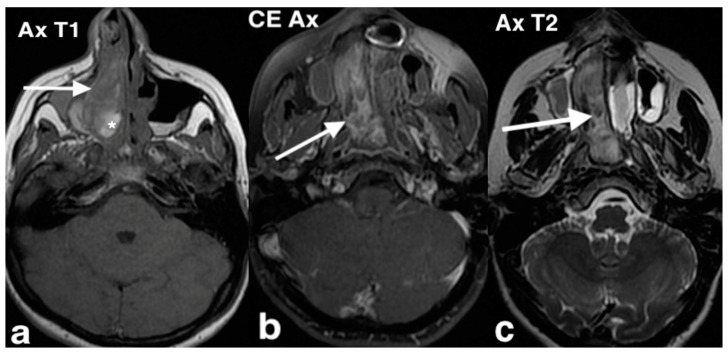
Hemangiopericytoma in a woman with epistaxis and bilateral nasal obstruction. On axial T1 MR image (a), the right nasal cavity is entirely occupied by an obstructing mass (arrow) with low–intermediate intensity and hyperintense hemorrhage areas (asterisk). Posteriorly, the mass, crossing the right choana, obstructs the nasopharynx lumen. On the axial fat saturation T1 after intravenous Gd image (b), the mass (arrow) is highly but inhomogeneously vascularized, with flow voids; on the axial T2 image (c), the mass (arrow) has medium–high intensity with an anterior low-intensity area, corresponding to hemorrhage; hypointense foci of flow void are also observed.
